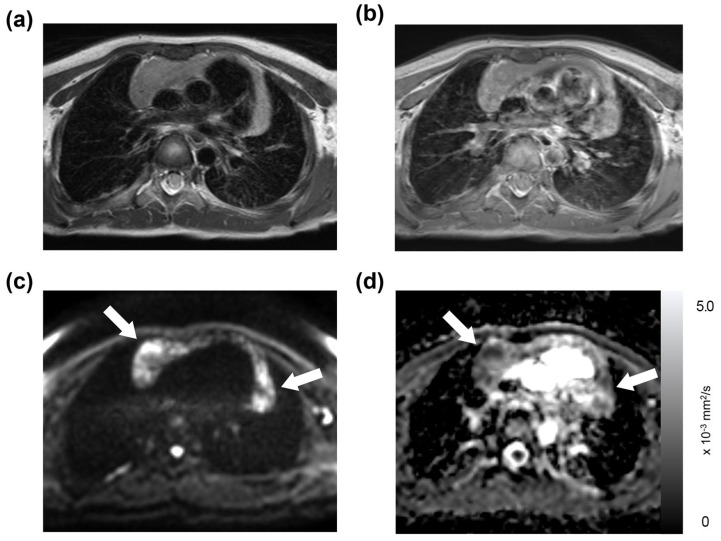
Multifocal restricted diffusion in the thymus: a 6-year-old boy with a history of mediastinal ganglioneuroblastoma. This patient underwent an MRI on a Philips scanner, with their laboratory data showing a WBC count of 5.08 × 10^3^/μL, an absolute neutrophil count of 1.97 × 10^3^/μL, an absolute lymphocyte count of 2.42 × 10^3^/μL, and a platelet count of 288 × 10^3^/μL. The thymus was found to be slightly hyperintense on both the T2-weighted (a) and T1-weighted images (b). Multifocal restricted diffusion was present on the DWI (c, arrows), which corresponds to low-signal intensity in the ADC map with a mean ADC value of 1.17 × 10^−3^ mm^2^/s (d, arrows).
